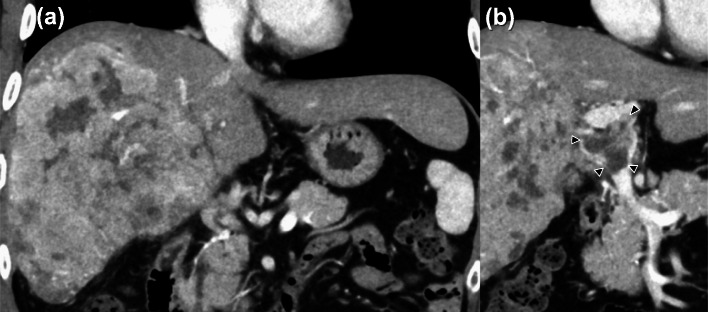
CT findings before proton beam therapy. a A hepatic tumor with a diameter of 16.0 cm is observed in the right lobe. b The tumor is accompanied by portal vein tumor thrombosis in the main trunk (black arrowheads)
